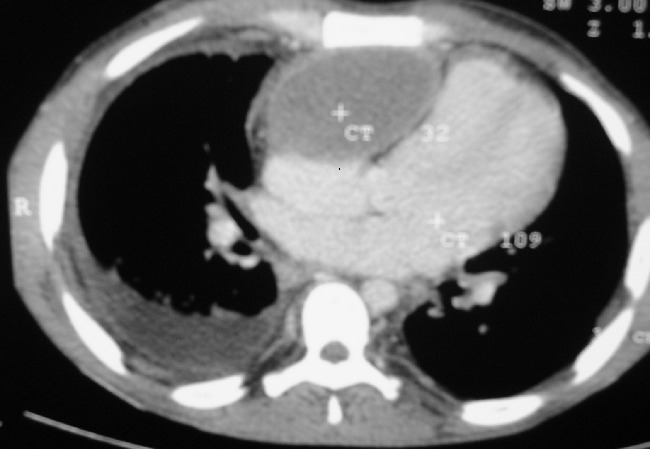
Axial CT image showing a retrosternal, anterior mediastinal fluid filled mass compressing the right ventricle and atrium. The mass has a well defined margin, non contrast enhancing and pushes RV to the left
